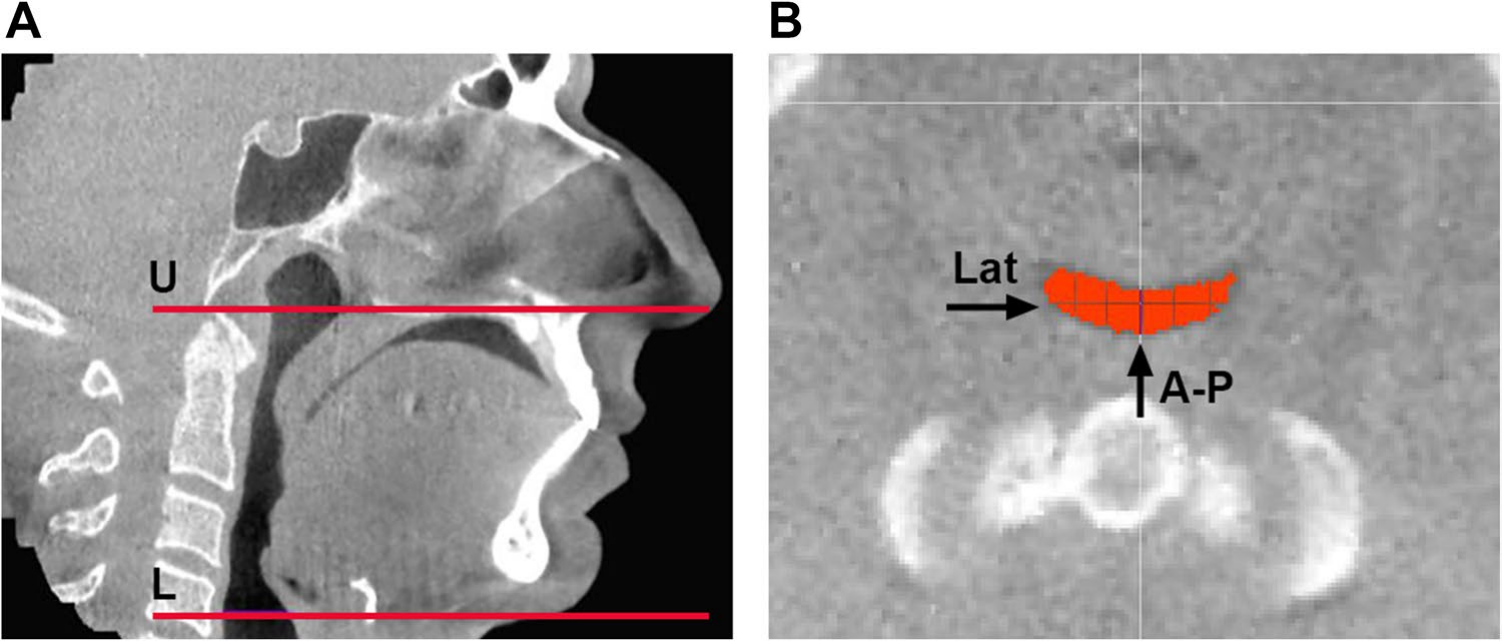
Upper airway boundaries and measurements of the upper airway shape, using cone beam computed tomography (CBCT) imaging. (A) The segmentation of the upper airway from the upper boundary (U) to the lower boundary (L) in the mid-sagittal plane. (B) The measurement of the anterior–posterior dimension (A-P) and lateral dimension (Lat) of the minimum cross-sectional area of the upper airway (CSAmin) in the axial plane
